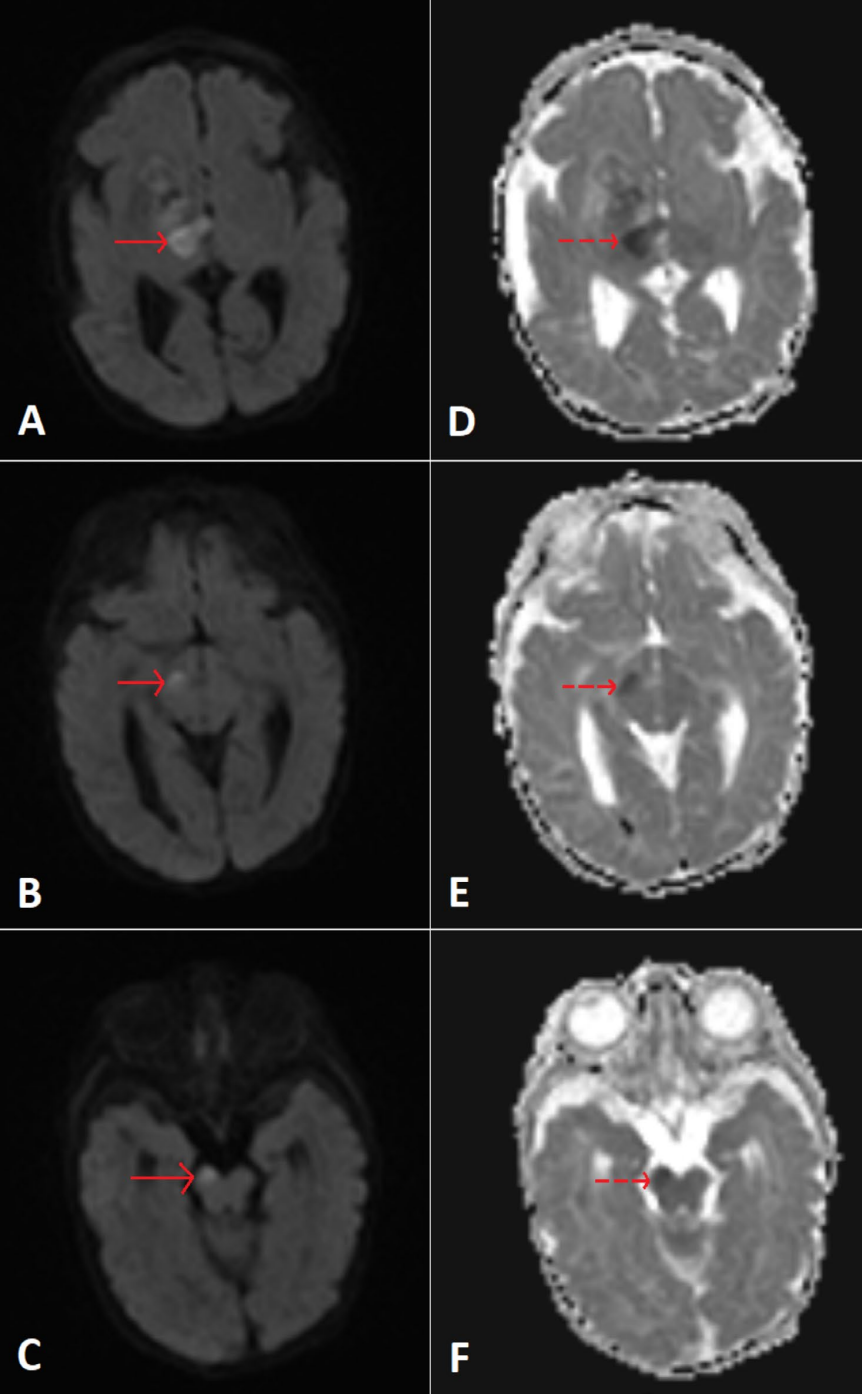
Axial diffusion‐weighted images for patient 1's brain (A, B & C: diffusion‐weighted images (DWI), D, E & F: apparent diffusion coefficient (ADC)). The images are noted showing true diffusion restriction (high DWI—red arrows—with corresponding low ADC value—dashed red arrows) involving the right subthalamic nucleus, the right anterior aspect of the mid‐brain and pons (right corticospinal tract), mostly representing Wallerian degeneration.
